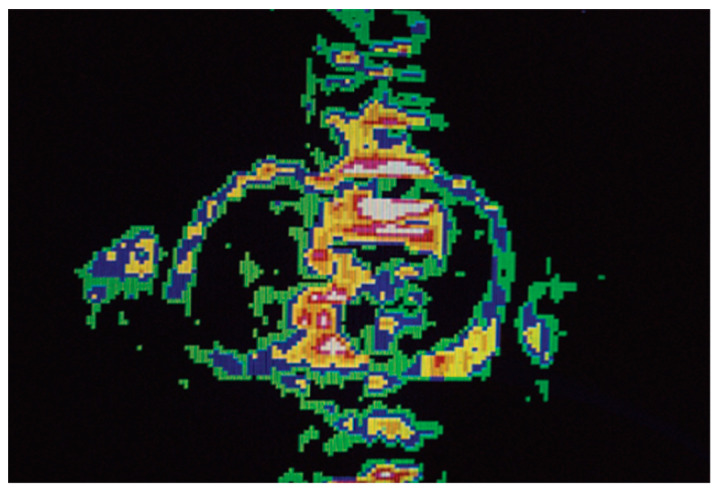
One of the first MRI images of the heart was acquired in a volunteer using the Aberdeen NMR imager in 1979, before the spin-warp breakthrough. Although the contours of the body and some internal structures can be recognized, severe artefacts caused by cardiac motion were present preventing its clinical use. Image courtesy of Bill Edelstein.
